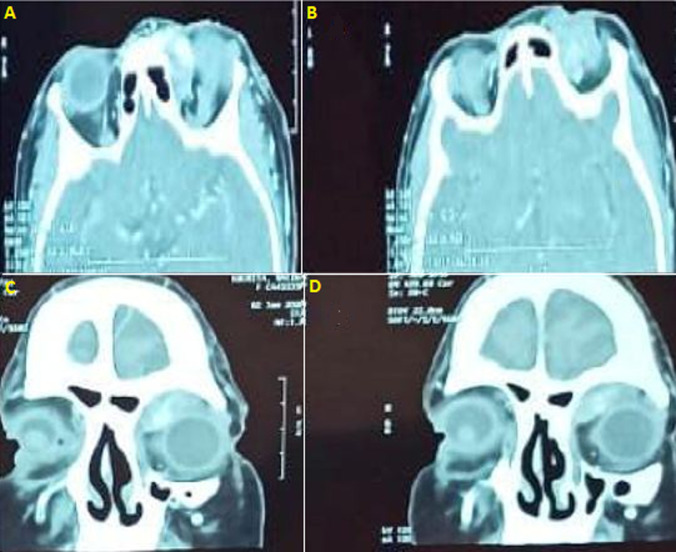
orbital CT scan with enhancement: (A,B) axial section; (C,D) coronal section, showing vascular lesion of the upper internal angle of left orbit
